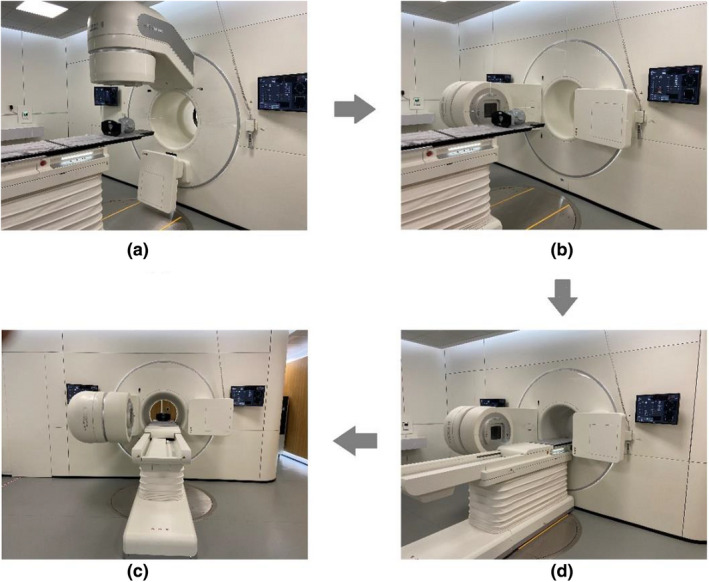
Room‐view photos of FBCT acquisition. (a) Patient is positioned at the treatment position. (b) Gantry is rotated to 270°, and meanwhile, (c) couch base is driven close to the gantry and couch top is translated to the CT bore. (d) FBCT scanning is started.
